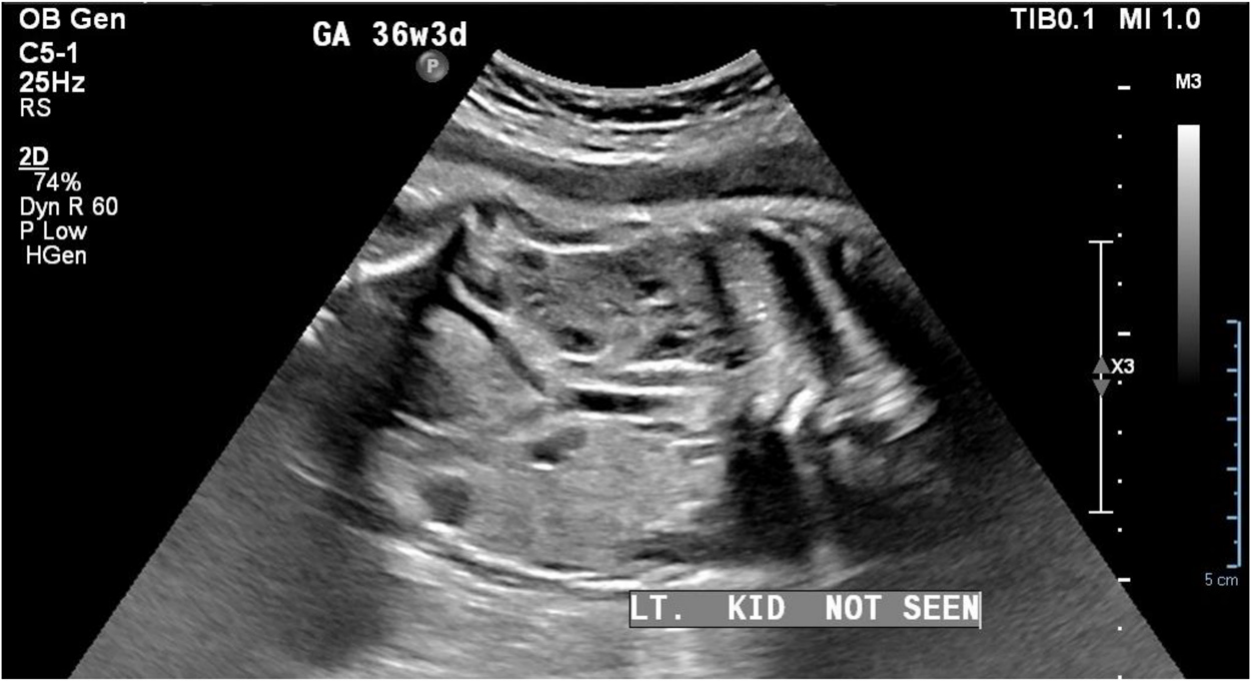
Antenatal ultrasound showing non-visualization of the left fetal kidney. The right kidney appears in its normal location.
